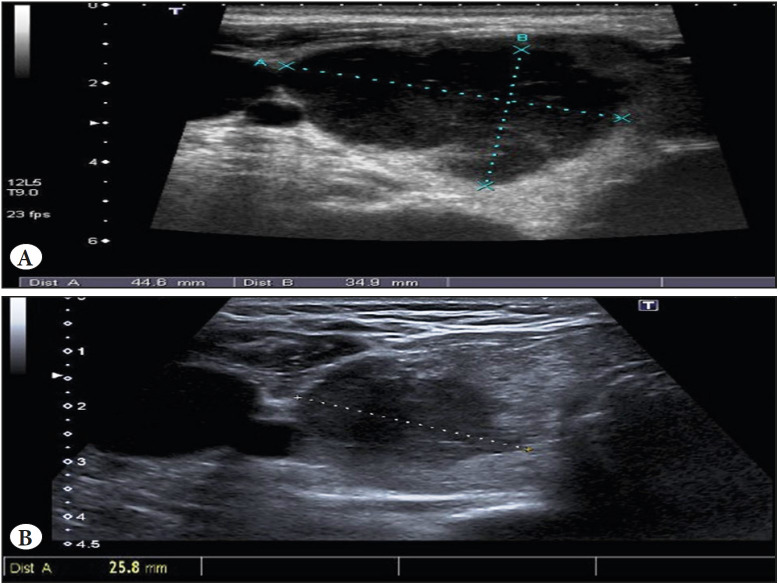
Right thyroid ultrasonography. The tests were performed two months apart. (upper: first ultrasonography). Note the tumor measures.
